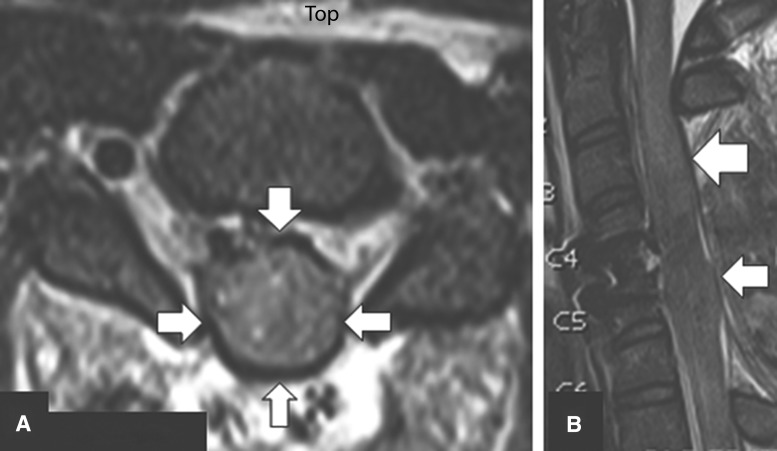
Post-operative axial (A) and midsagittal (B) MRI images of a motor complete traumatic spinal cord injury indicating severe spinal cord swelling with no visualization of subarachnoid space in the region of laminectomy from C3-C6 (arrows).
